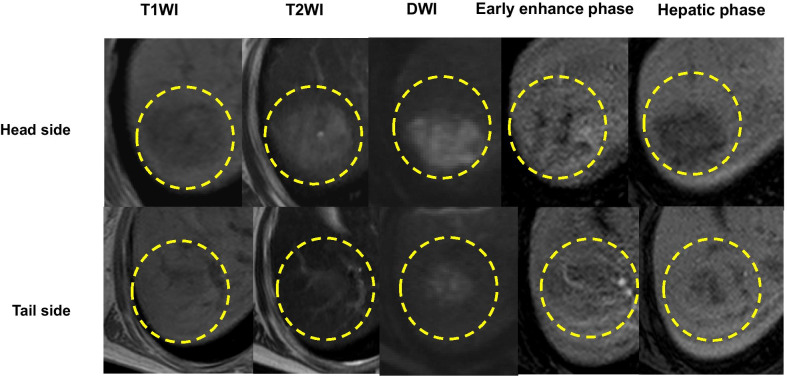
Magnetic resonance imaging (MRI). MRI revealed a tumor in liver segment 8 with low- to iso-intensity on T1-weighted imaging (T1WI), high- to iso-intensity on T2-weighted imaging (T2WI), high intensity on diffusion-weighted imaging (DWI) and low intensity on hepatocellular images, and the enhanced pattern was the same as that of computed tomography (dotted circle)
